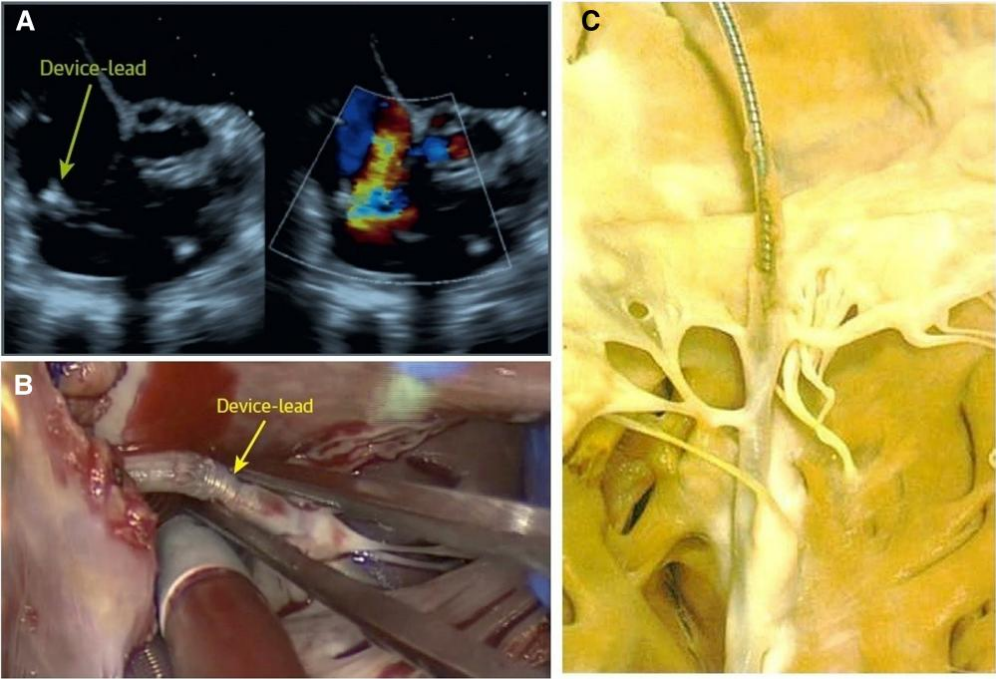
Surgical situ in a patient with lead-related tricuspid regurgitation. (*A*) Device lead attached to the tricuspid valve in echocardiography; (*B*) intra-operative view of an attached cardiac implantable electronic device lead; and (*C*) device lead attached to the tricuspid valve and the subvalvular apparatus with significant ingrowth.
